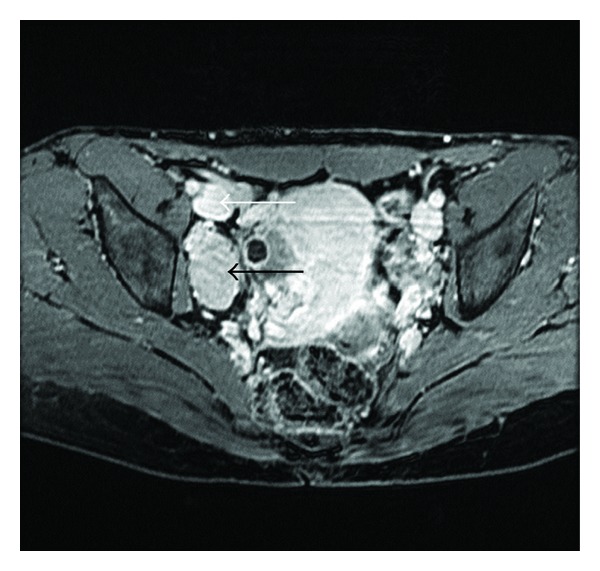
Pelvic magnetic resonance imaging: axial GE-T1 fat sat with gadobenate dimeglumine in venous phase shows intense enhancement (black arrow), similar to the vessels (white arrow).
